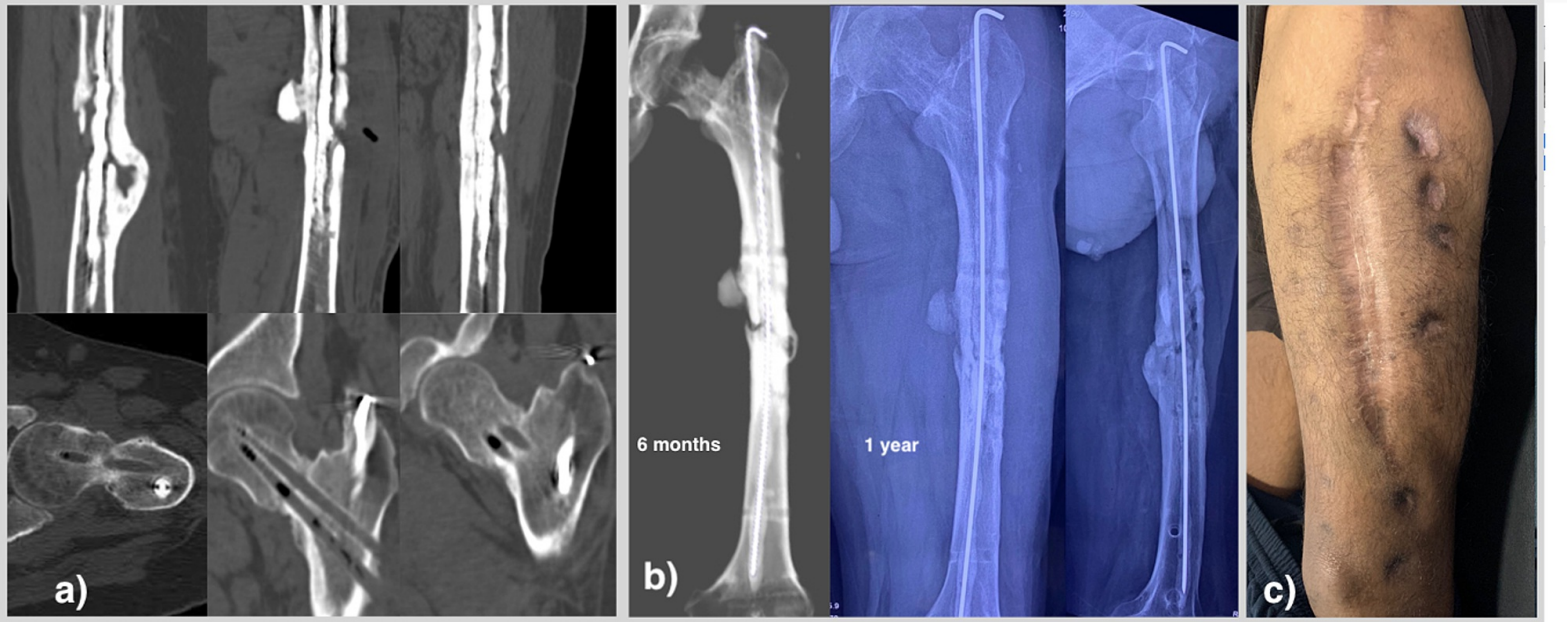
a) The CT images at the 6-month postoperative period suggest at least three cortices united for the femoral shaft and complete union of the femoral neck. b) The 6 month and one-year follow-up radiographs of the same patient showing consolidation of both fracture sites (femoral neck and femoral shaft). c) The clinical picture showing healing of all wounds and pin sites.
